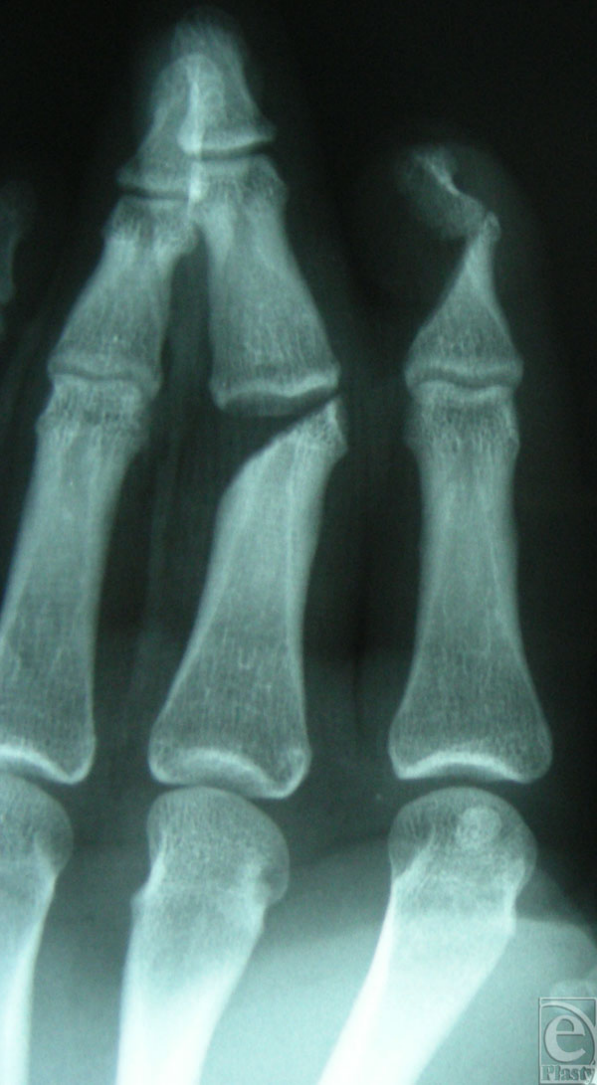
Anteroposterior radiograph showing the extension of articular destruction by the planer machine in the DIP II and PIP III joints.
